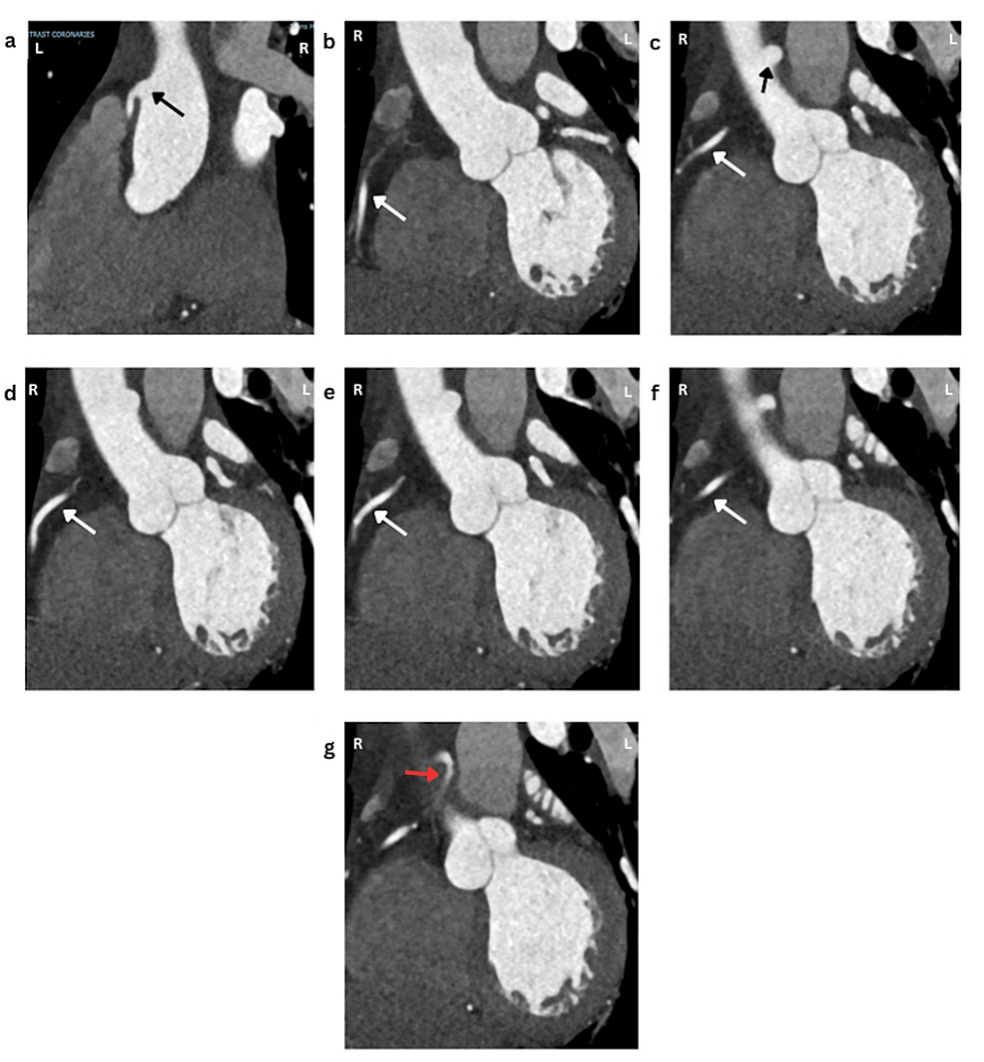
Computed tomography coronary angiogram revealing an anomalous right coronary artery originating superior to the left sinus of Valsalva. (a) The high take-off from the left aortic arch (black arrow). (b-f) The anomalous right coronary artery course (white arrow). (g) A brief interarterial course of the anomalous right coronary artery (red arrow). R: right; L: left
